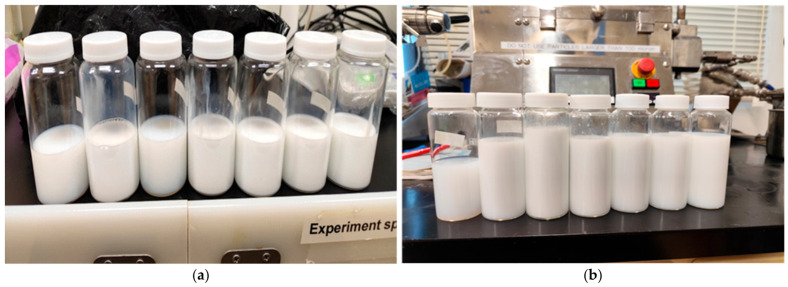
(a). Ultrasonication method on Day 01 and (b). High-pressure method on Day 01.
